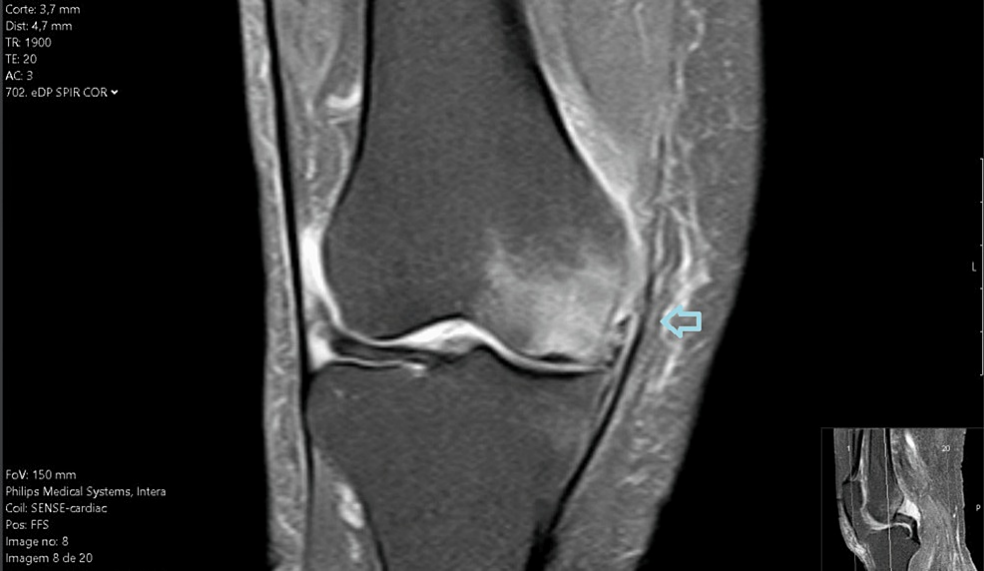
MRI of the right knee in the coronal plane presenting osteochondral lesion of the internal femoral condyle.
